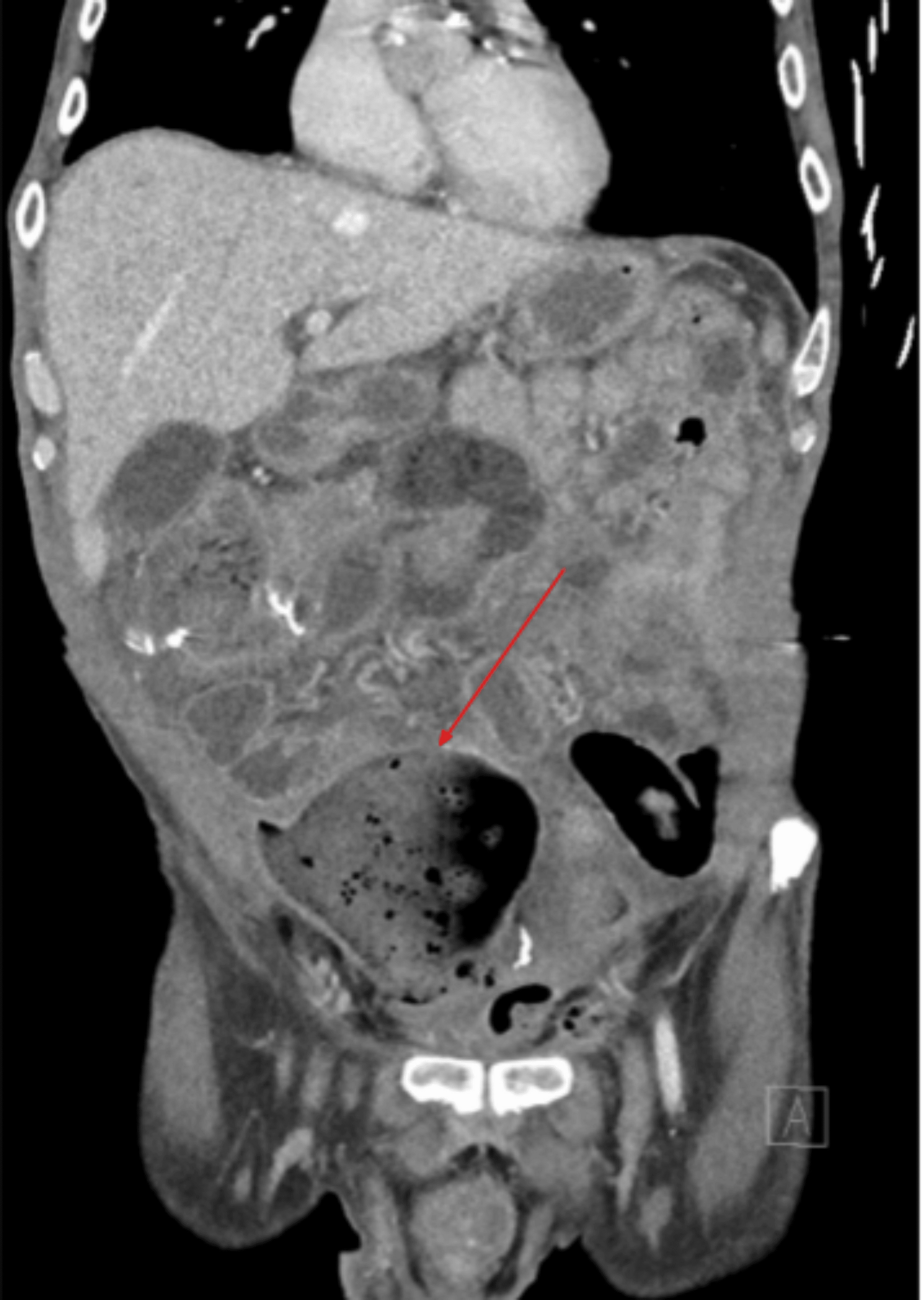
CT abdomen/pelvis in coronal view demonstrating a large collection with an air-fluid level and feculent material (red arrow) in the right lower abdomen and pelvis CT: Computed tomography
